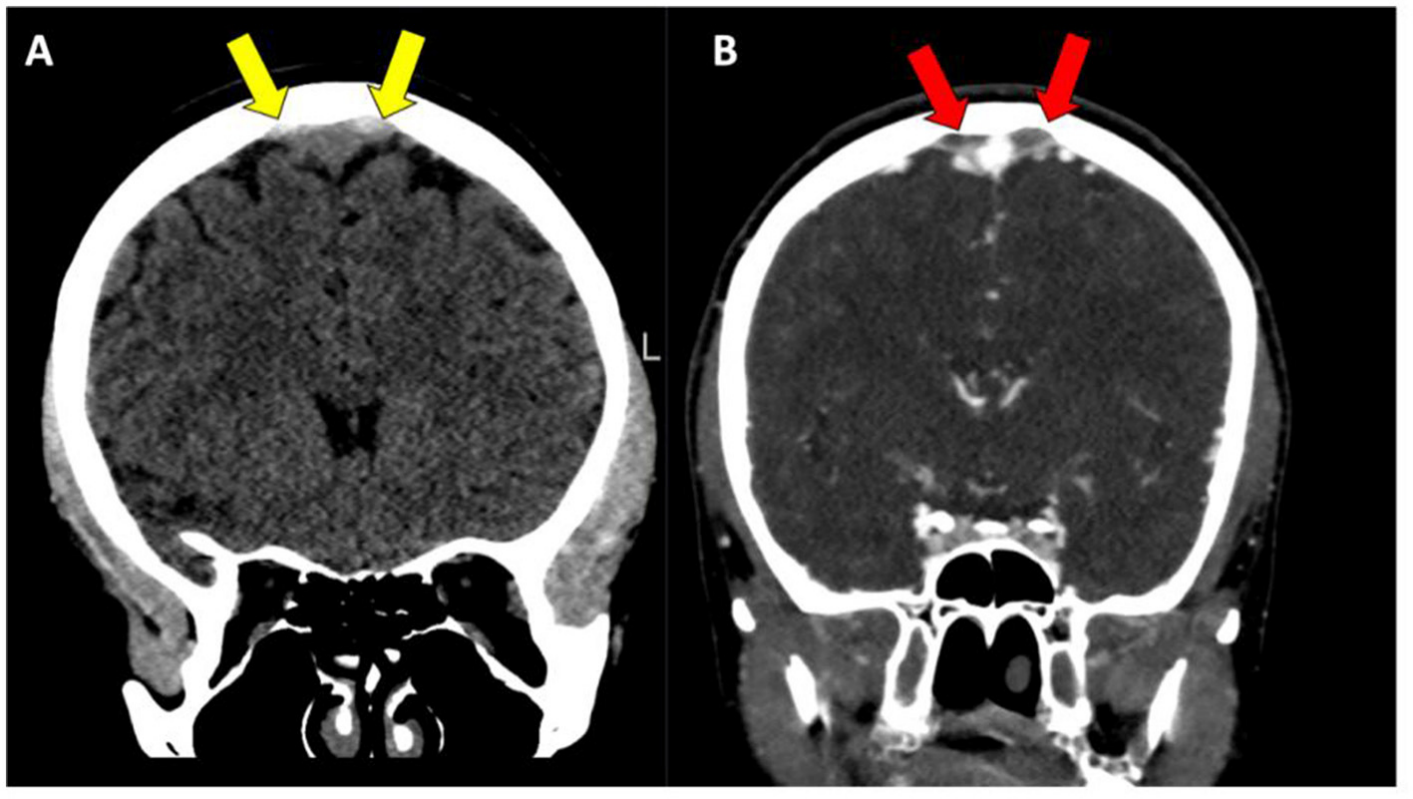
(A, B) Patient with parasagittal intracranial venous lake thrombosis (IVLT), with high attenuating thrombosis located on both sides of the superior sagittal sinus on non-contrast enhanced CT images (yellow arrows), with a corresponding lack of filling in the same venous lake structures on CT-venography (red arrows).
